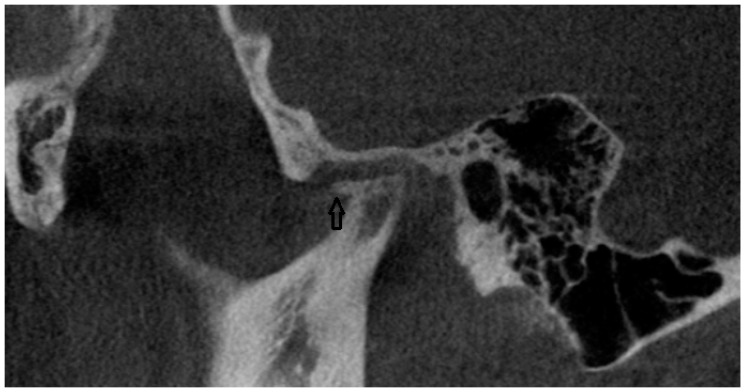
CBCT scan (black arrow points to osteophyte).
